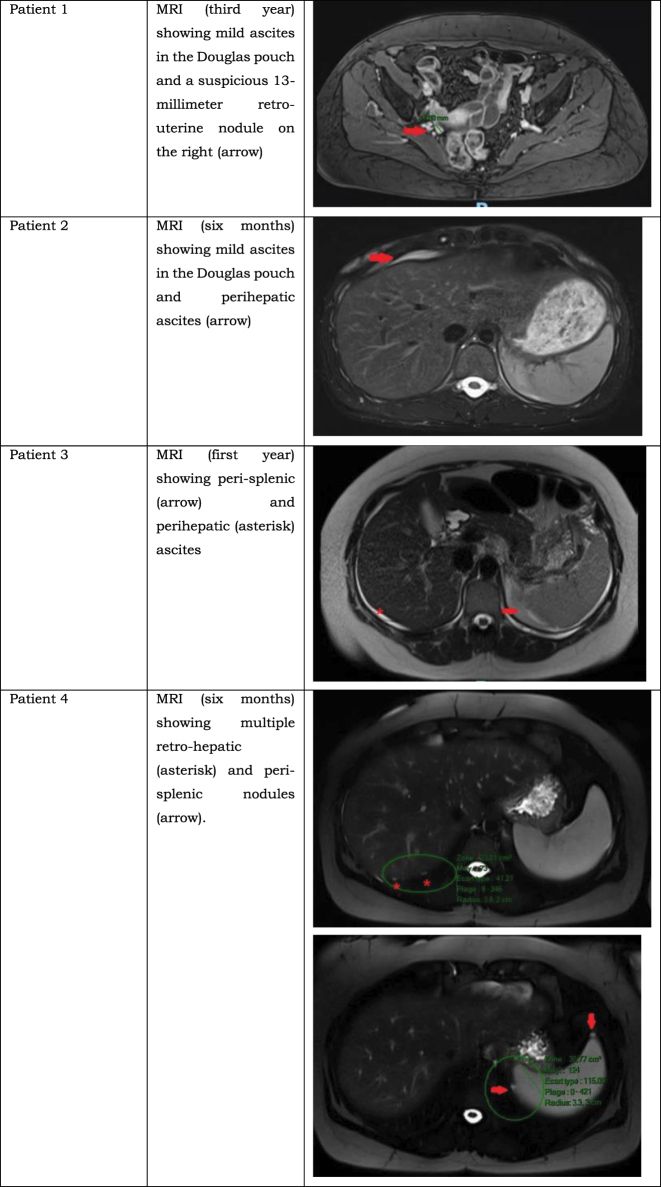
Suspicious lesions seen on surveillance MRI suggesting disease progression.
